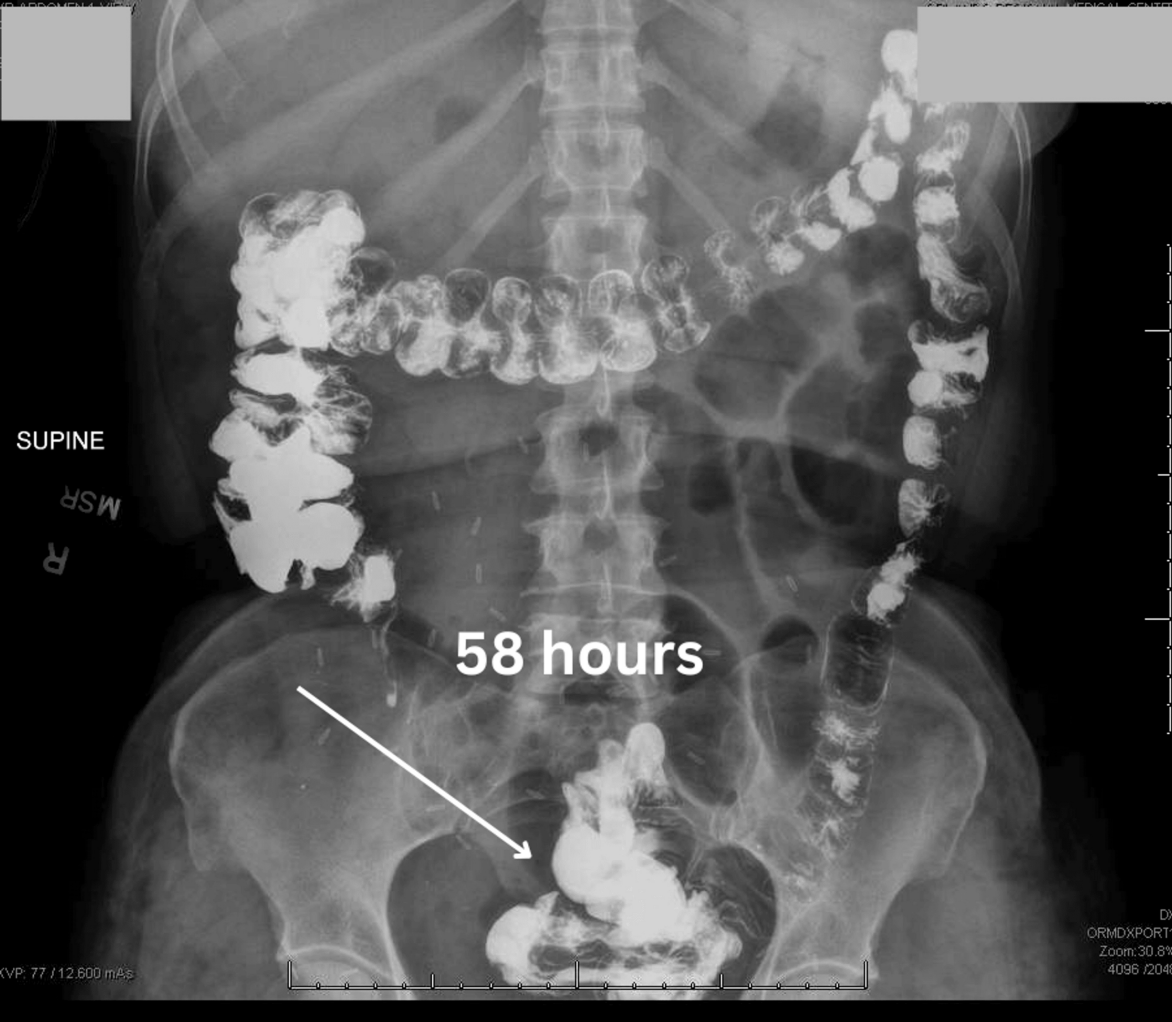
Abdominal radiograph demonstrating barium contrast in the distal colon at 58 hours after the start of the small bowel follow-through
